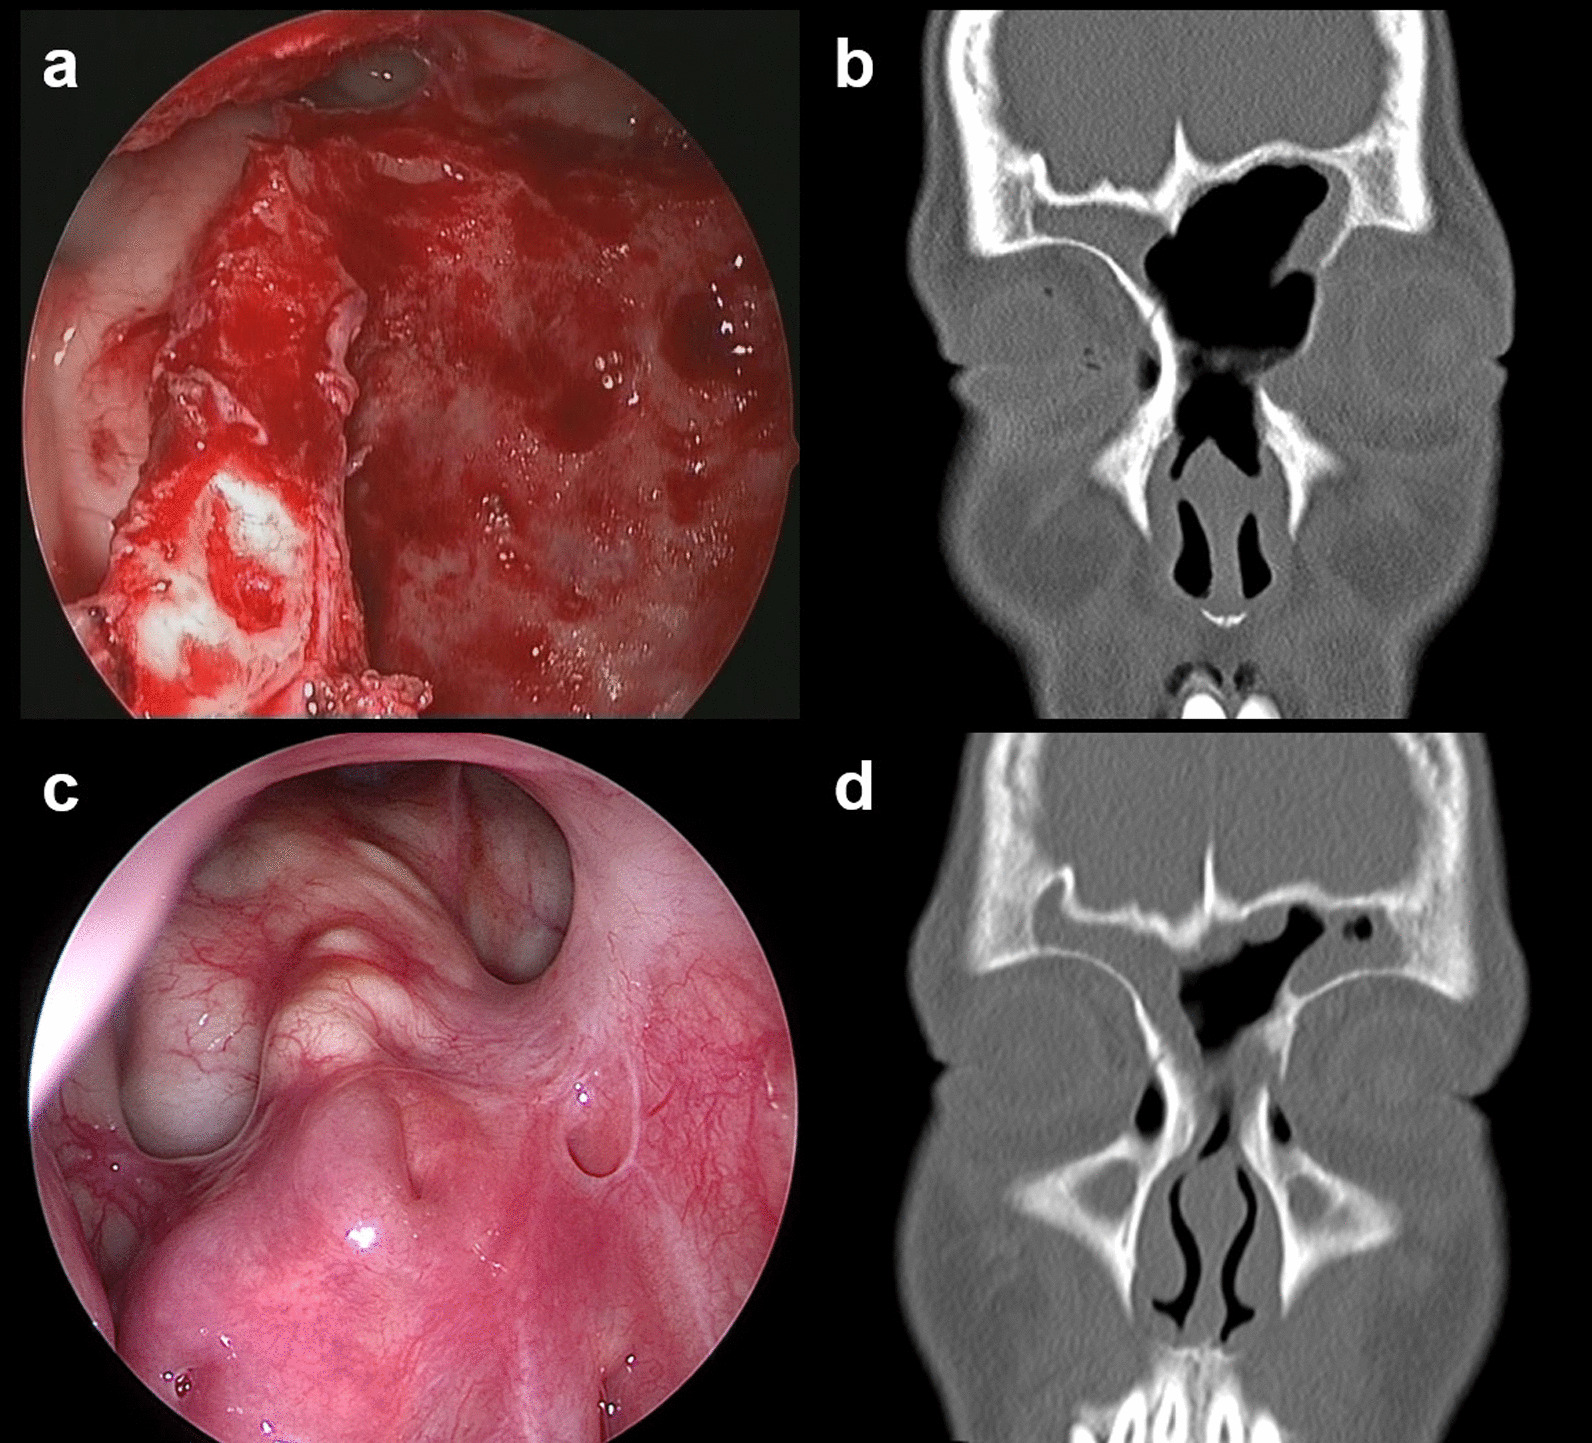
Postoperative follow-up images of ossifying fibroma of the frontal sinus. a Endoscopic images after complete tumour resection via the extended Draf IIb procedure, b postoperative nasal sinus coronal and axial computed tomography (CT) scans indicating complete tumour resection, c 4-year postoperative follow-up endoscopic images, d 4-year postoperative nasal sinus CT re-examination indicating no tumour recurrence
